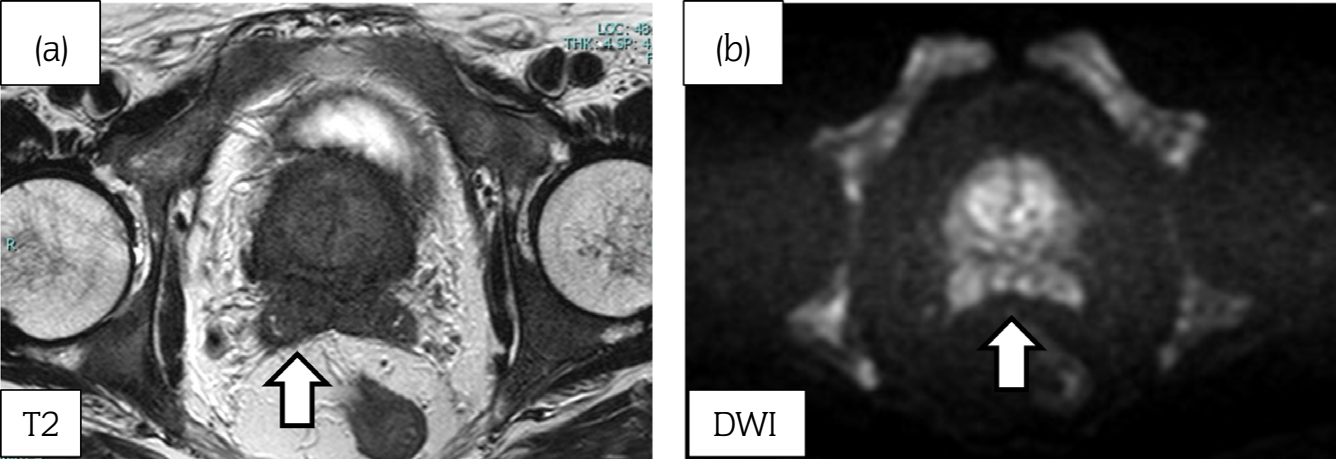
MRI of the prostate showed an indistinct hypointensity on T2‐weighted images (a) with a focal area of diffusion restriction. (b).
